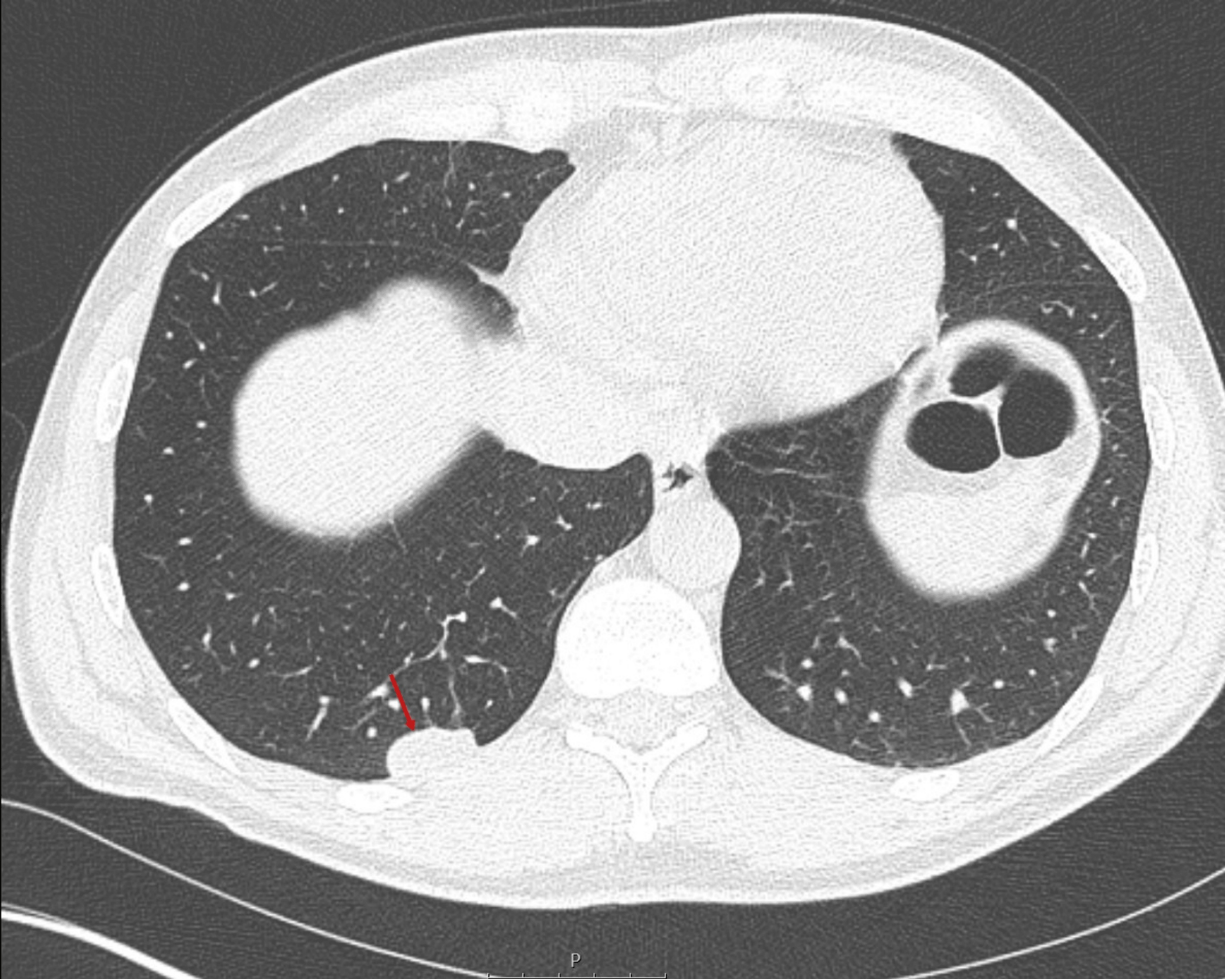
Coronal CT image showing a large flattened nodule in the right lower lobe
